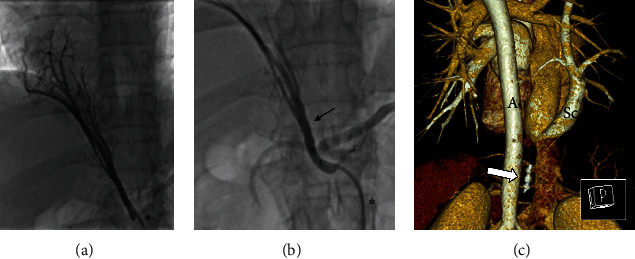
Embolization of a pulmonary sequestration artery (PSA) by AVP-2 (8 mm; arrows) in case 34. (a) PSA derived from the abdominal aorta (angiography). (b) Residual shunt after embolization (angiography). (c) Total PSA occlusion in computed tomography angiography before discharge (Ao = aorta; Sc = scimitar vein). Endovascular catheters are marked by asterisks.
